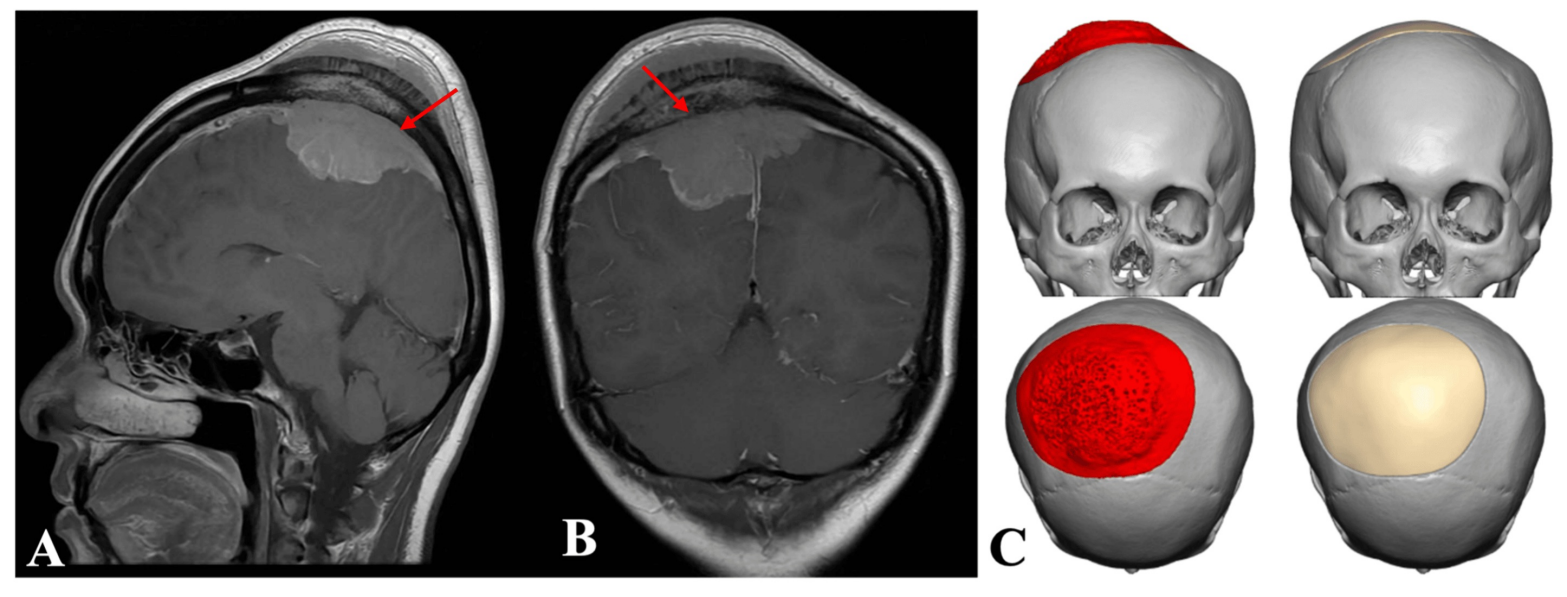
(A) Sagittal and (B) coronal preoperative images of MRI brain (T1-weighted with contrast) showing right parasagittal tumor (red arrow). (C) Preoperative implant design MRI: magnetic resonance imaging
